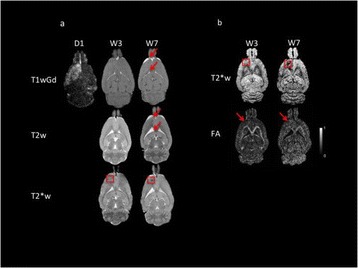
a T1wGd, T2w, T2*w MRI (3.0 T), and b T2*w and DTI MRI (9.4 T) of a representative brain after 6 weekly sonications
